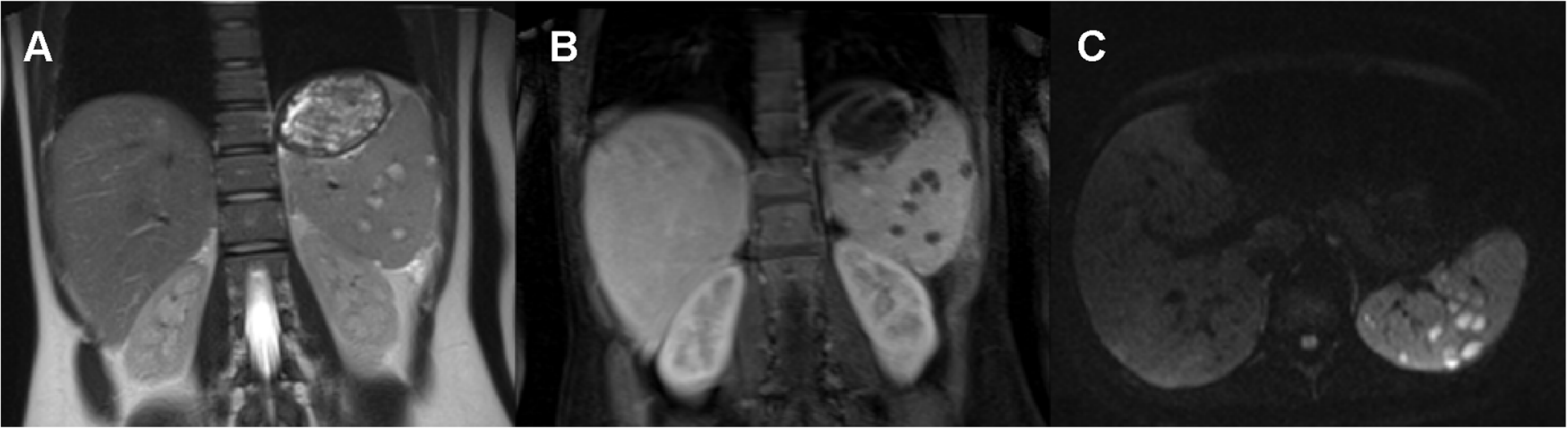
Follow up MRI of the abdomen (24 days after admission). A, the coronal T2w image shows hyperintense lesions with hypointense rim in the spleen. B and C, the coronal postcontrast T1w image and transverse DW image (b 800 sec/mm^2^), show an increased number and size of the lesions.
